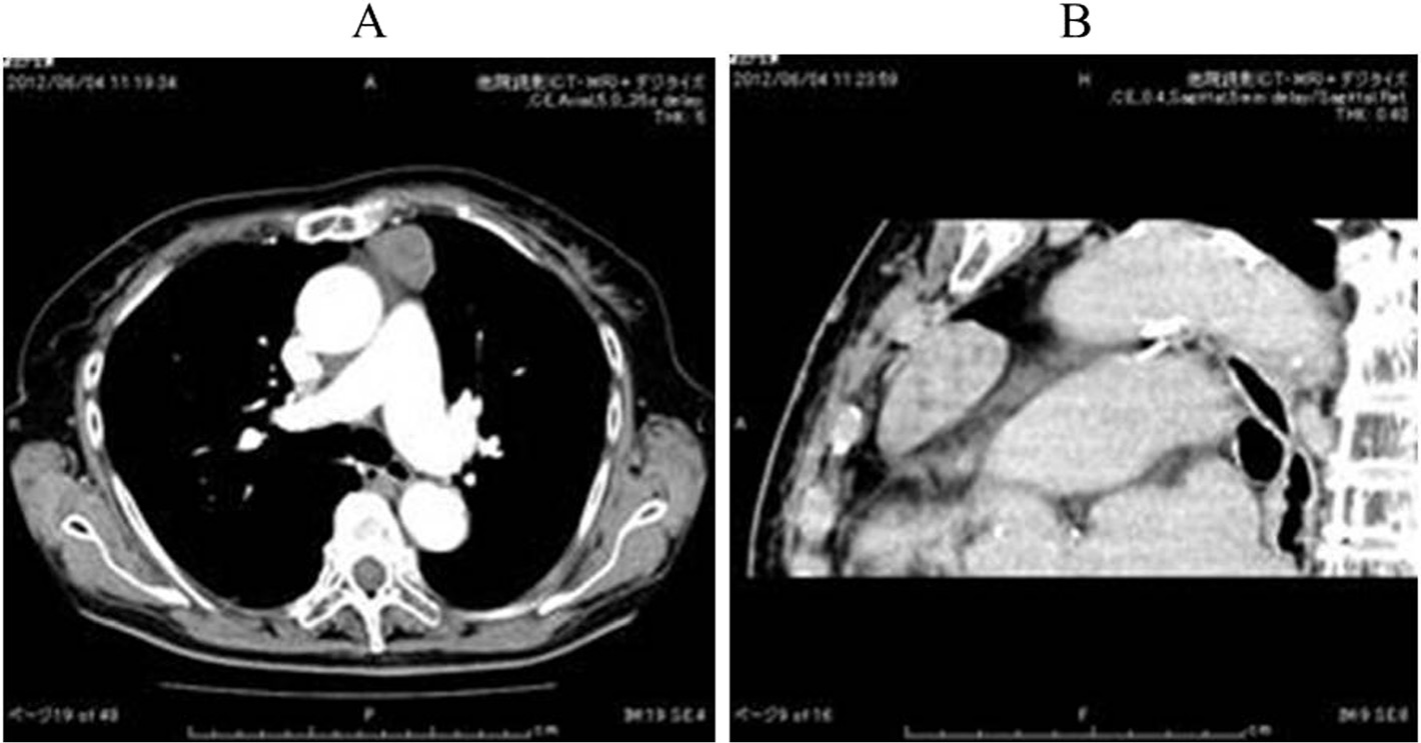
Preoperative chest computed tomography (CT) scan demonstrated a homogenous, sharply circumscribed mass in the anterior mediastinum. (A): Enhancement showed a mass that was not enhanced. (B) Sagittal view.
